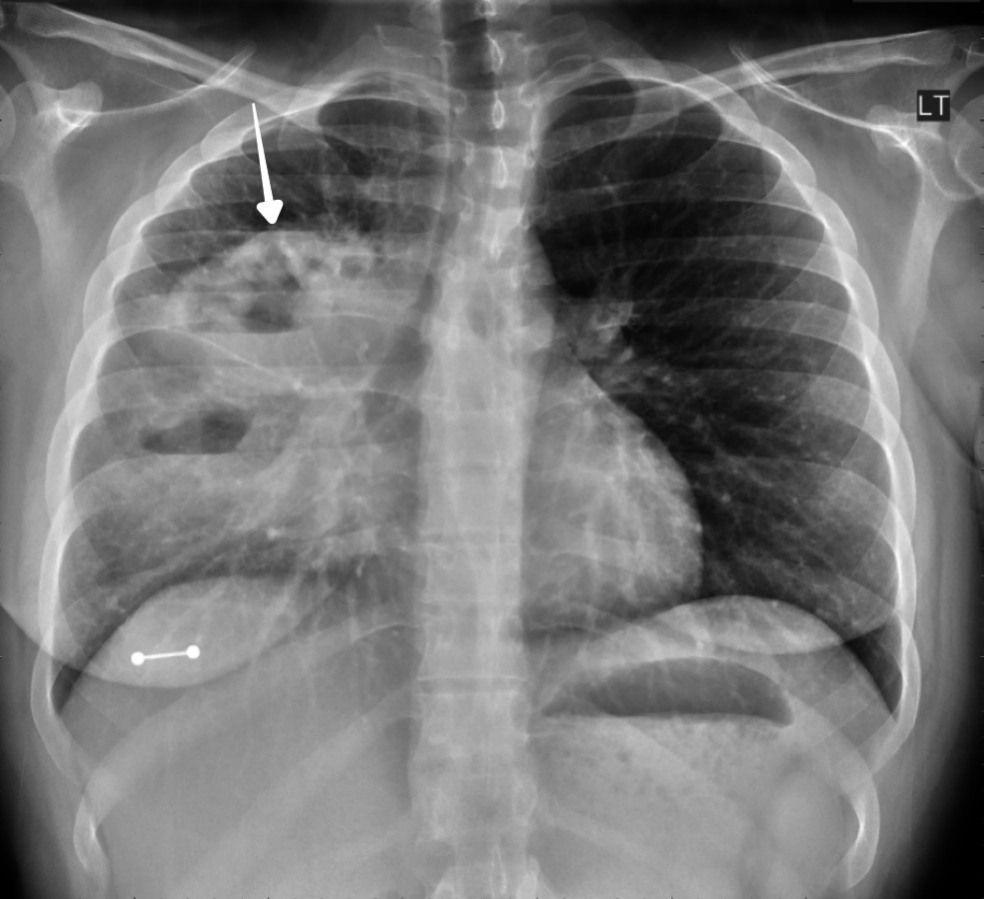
Chest X-ray Chest X-ray (CXR) showing multiloculated cavitary lesions in the right upper and middle lobes, indicated by the white arrow.
